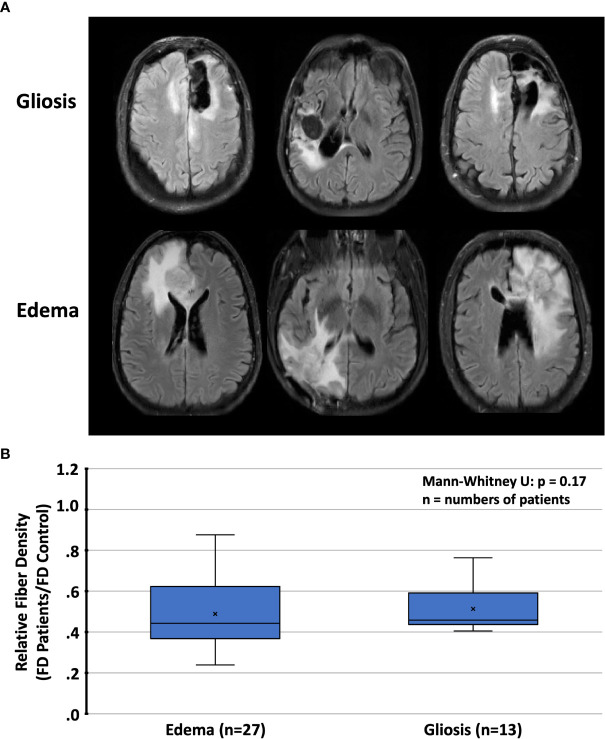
(A) Representative cases with T2/FLAIR hyperintense regions and (B) relative fiber densities (FD) in patients with radiation-induced gliosis or peritumoral edema. T2/FLAIR, T2-weighted fluid-attenuated inversion recovery.
